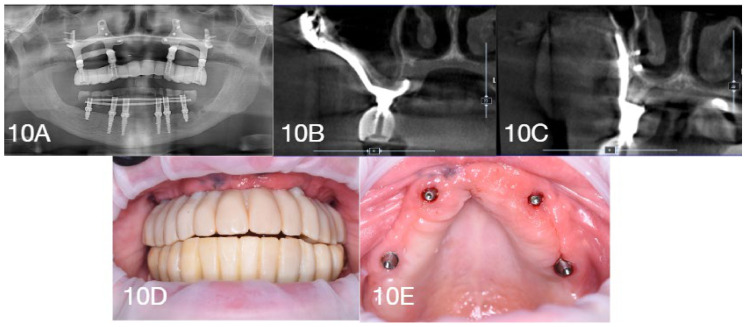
Two-year clinical and radiographic follow-up (Case 1). (A) Follow-up orthopantomogram showing the structural integrity of the bilateral full-arch subperiosteal implant. (B,C) CBCT cross-sections confirming absolute congruence and the absence of gaps at the bone-implant interface. (D) Final aesthetic and functional integration at 24 months. (E) Intraoral view demonstrating healthy peri-implant mucosa free from signs of inflammation or peri-implantitis.
